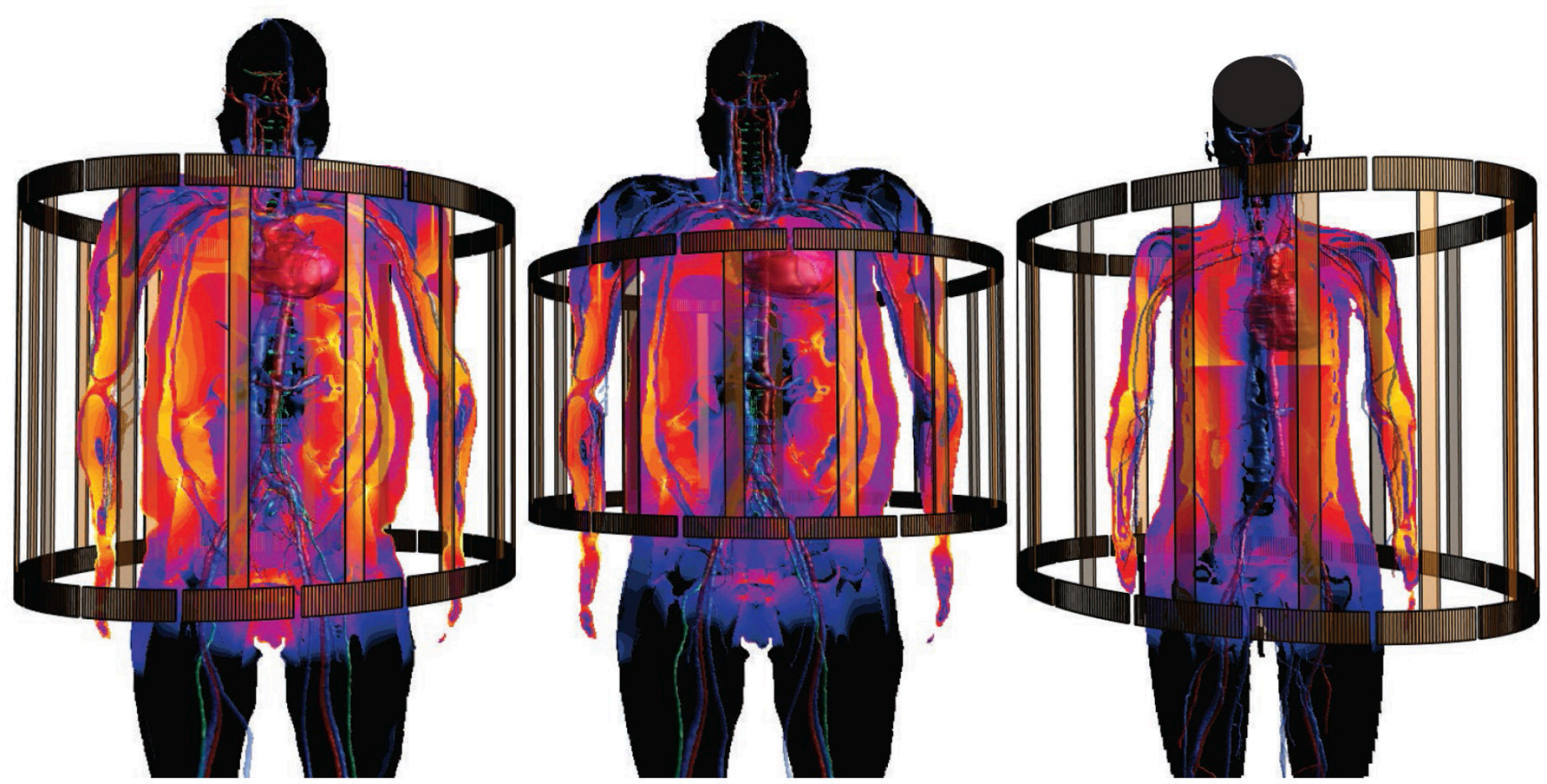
Anatomical models functionalized with incident *in vivo* field conditions from MRI RF-coil exposure, for use in MRI implant safety assessment (s. section 2.2.4). Different coils and anatomical models are shown.
